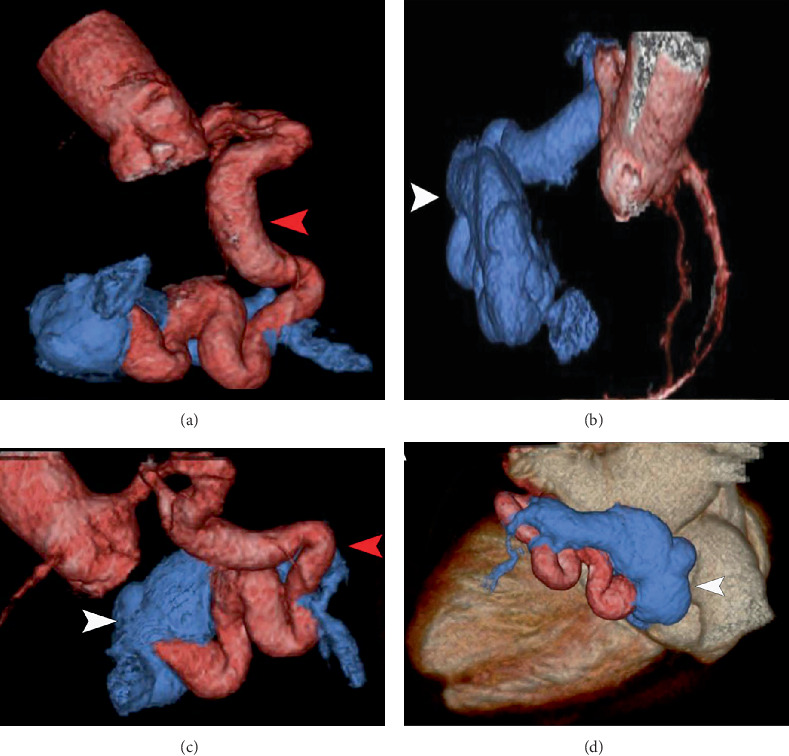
(a–d) 3D reconstruction of coronary CT angiogram showing an ectatic left circumflex artery (red arrow) and fistula to the coronary sinus (white arrow).
